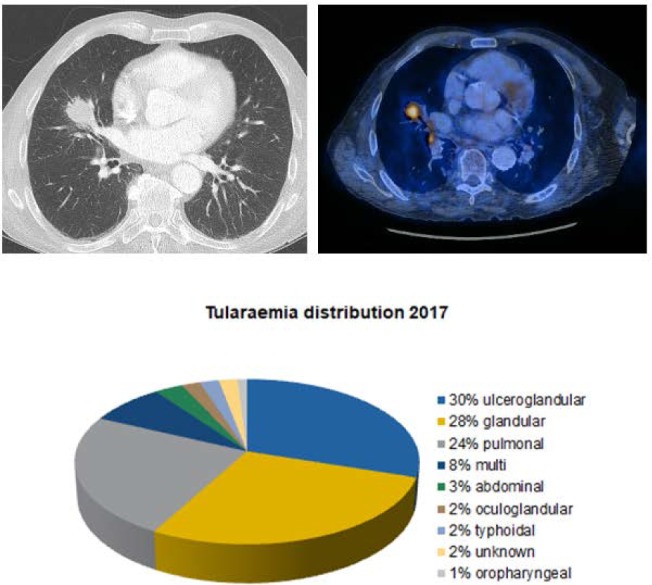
CT scan an PET scan showing a mass 3.4 cm in diameter in the right middle lobe and avide lymph nodes
